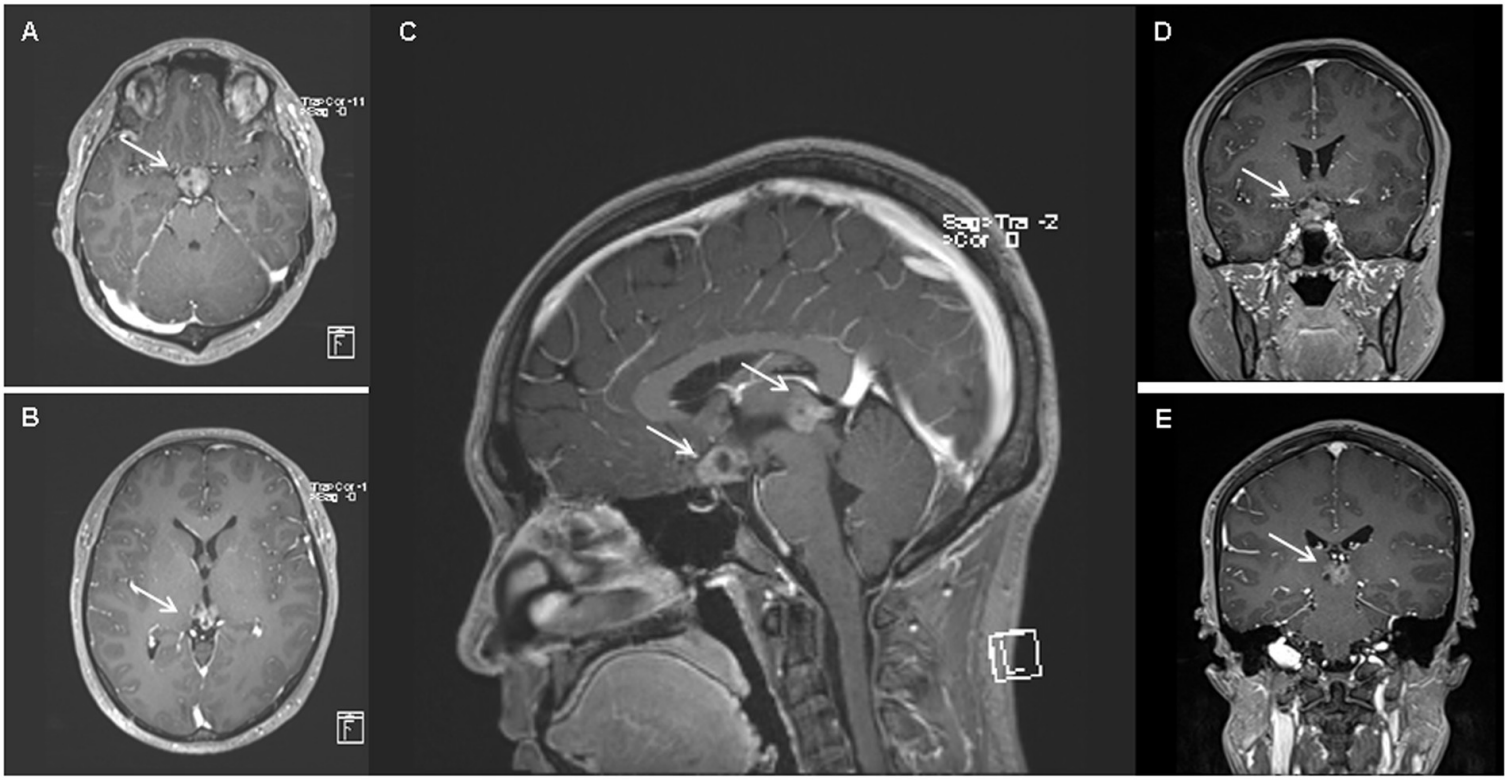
A case of bifocal germinoma at diagnosis. (a) Suprasellar lesion (white arrow) on MRI T1 gadolinium axial image. (b) Pineal lesion (white arrow) on MRI T1 gadolinium axial image. (c) Bifocal lesions (white arrows) on MRI T1 gadolinium sagittal image. (d) Suprasellar lesion (white arrow) on MRI T1 gadolinium coronal image; E) Pineal lesion (white arrow) on MRI T1 gadolinium coronal image.
